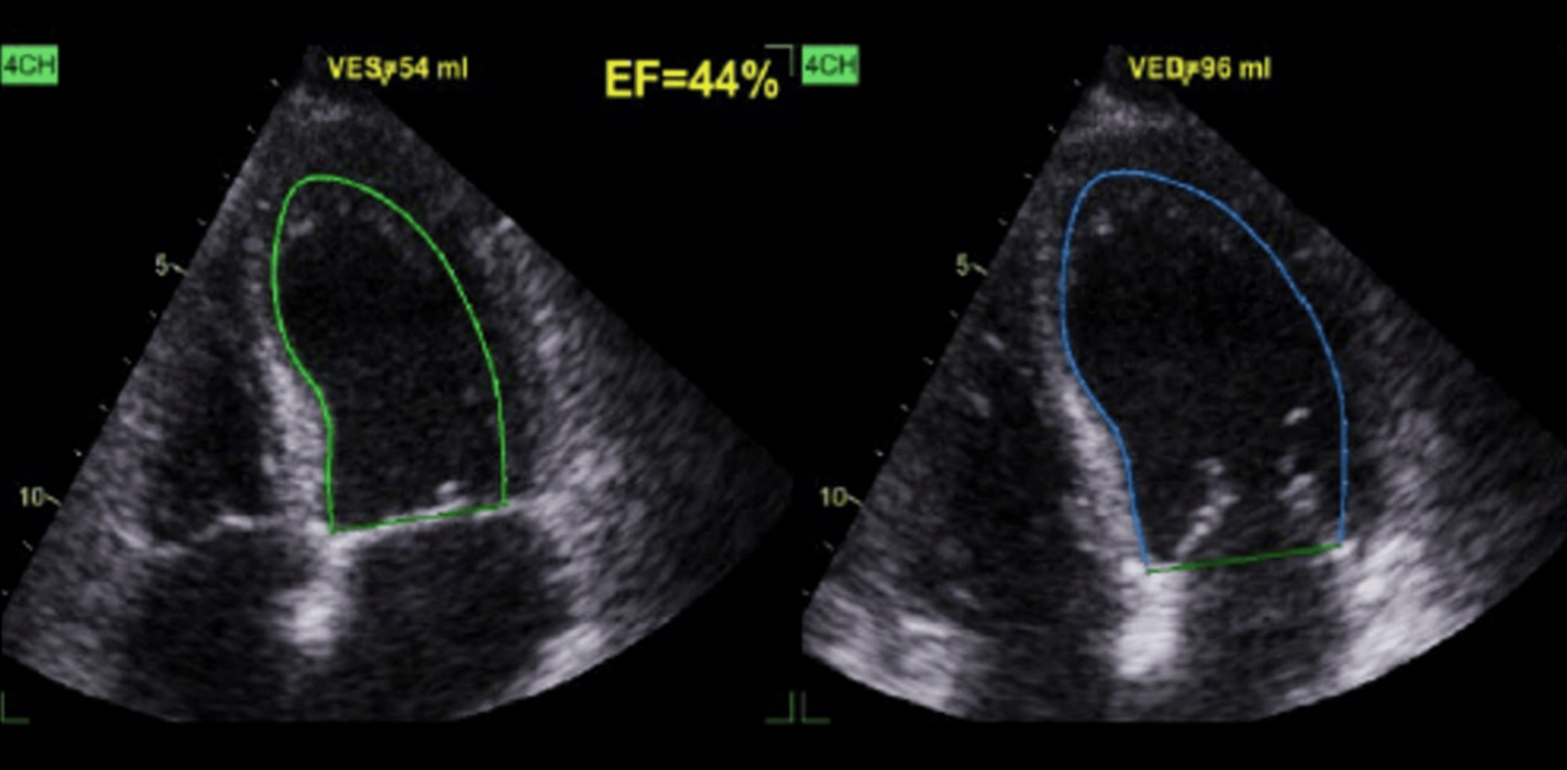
Thoracic echocardiography (four-chamber view) of the patient. The patient's thoracic echocardiography reveals a mildly reduced ejection fraction without any structural cardiac abnormalities. The image highlights the EF measurement. EF, ejection fraction; VED, volume end-diastole; VES, volume end-systole
